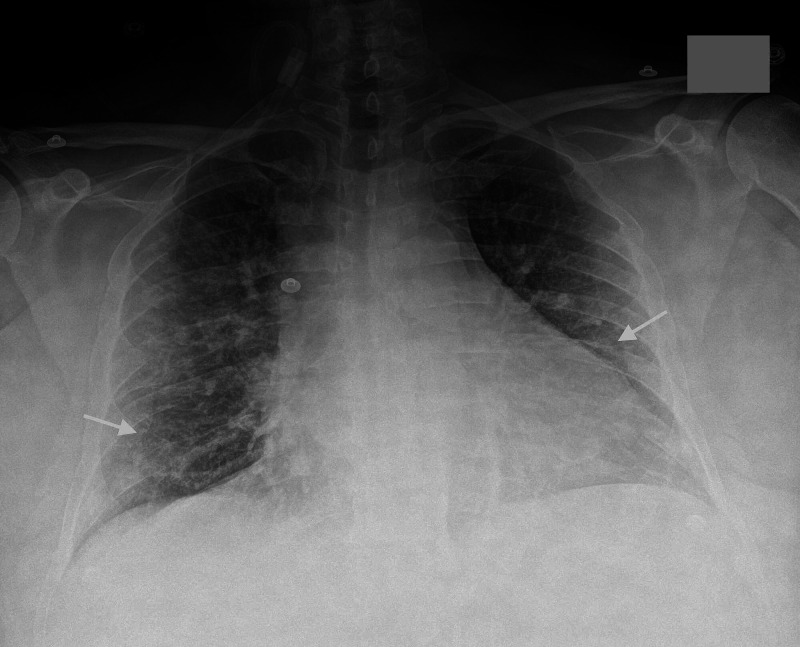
Chest radiography. Chest radiograph showing bilateral diffuse opacities more prominent in the lower lobes (arrows).
